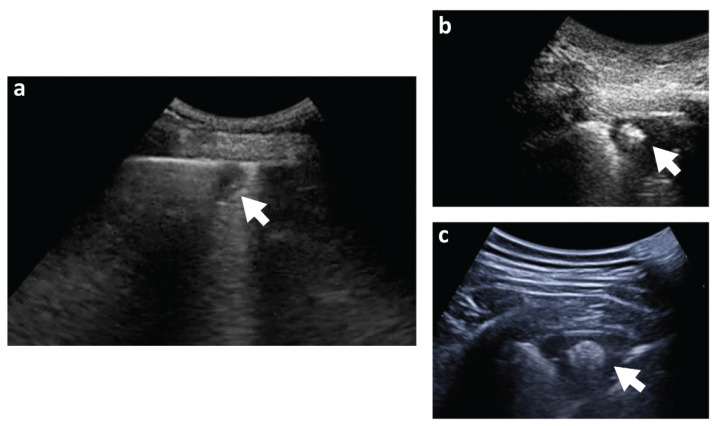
(a) Small (11 × 9 mm) subpleural consolidation detected at lung echography. Clear pleural discontinuity with the underlying hypoechoic area. (b,c) Typical ultrasound appearance of “bubbly consolidation” with a roundish hyperechoic area in the context. Arrows indicate the consolidation at lung echography.
